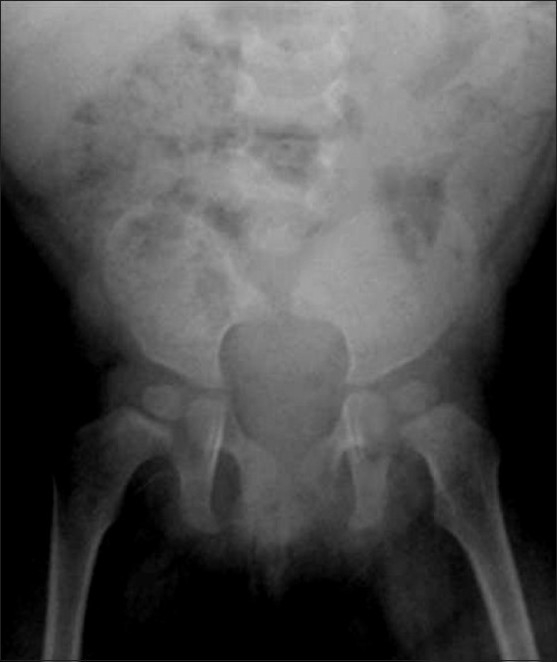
Caudal regression syndrome (complete agenesis of sacrum): Frontal radiograph of the pelvis shows a contracted pelvis due to absence of the sacral segments; the two iliac wings can be seen pseudo-articulating with each other and with the caudal portion of L3 due to the absence of the L4 and L5 vertebrae
